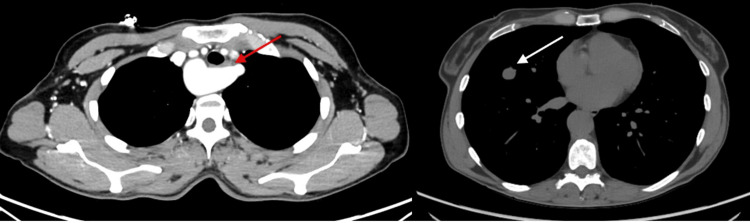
Angio-CT: right aortic arch vascular anomaly. Red arrow: the esophagus appears to be imprinted by the subclavian artery lusoria. White arrow: middle lobe nodule.
